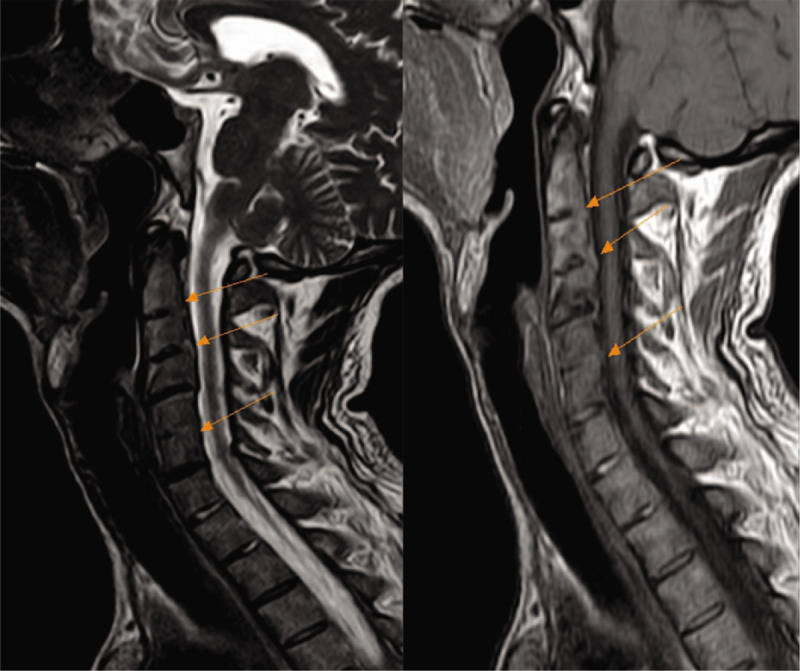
Spine magnetic resonance imaging (MRI) showed that the whole spine curvature became straight and changes of double sacroiliac joints were as follows. The changes include fusion of 2/3, 5/6 intervertebral space and mild posterior herniation of cervical 4/5, 6/7 intervertebral discs.
